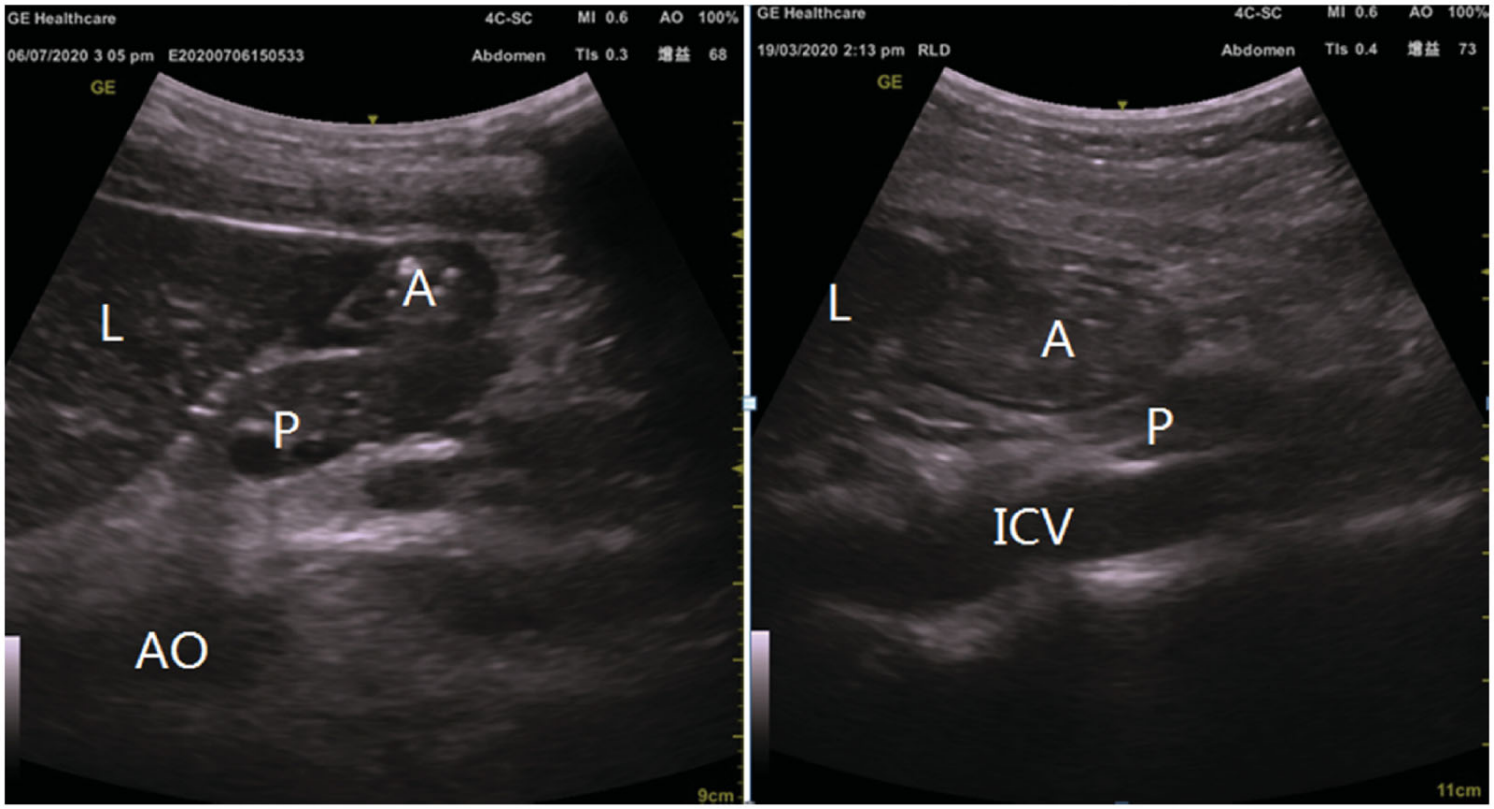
Ultrasound images. Representative ultrasound images showing a gastric antrum in the supine position (A) after overnight fasting and (B) at 5 min after a meal. A: antrum; L: liver; P: pancreas; IVC: inferior vena cava.
